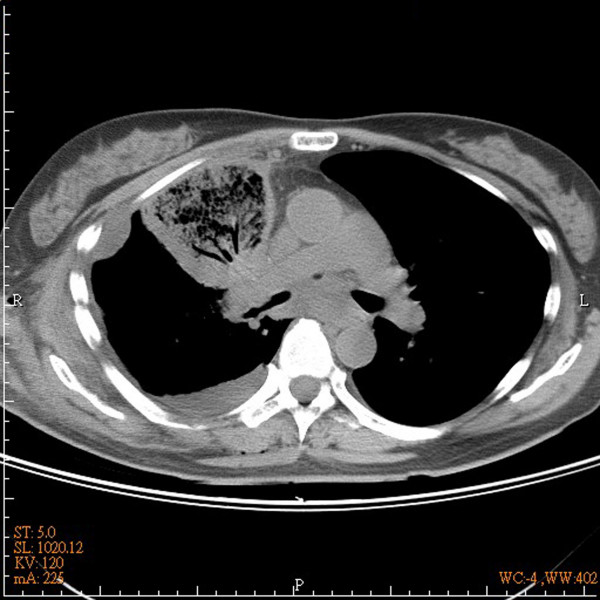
CT scan showed collapse and hemorrhagic consolidation in right middle lobe.
